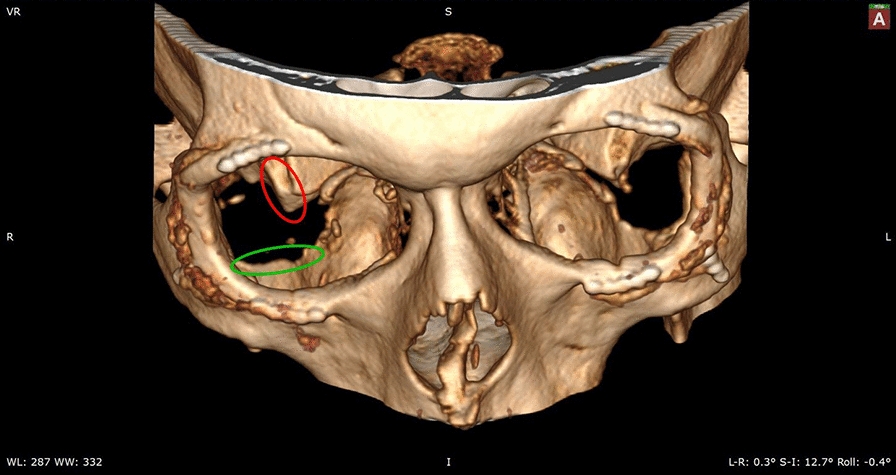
Visualization of post-orbital decompressions surgery CT. The post-orbital decompressions surgery CT (patient 2) shows insufficiently resected key point 1 on the right side (red circle) vs. well-resected key points on the left site. The green circle on the right side indicates the well resected key point 2
